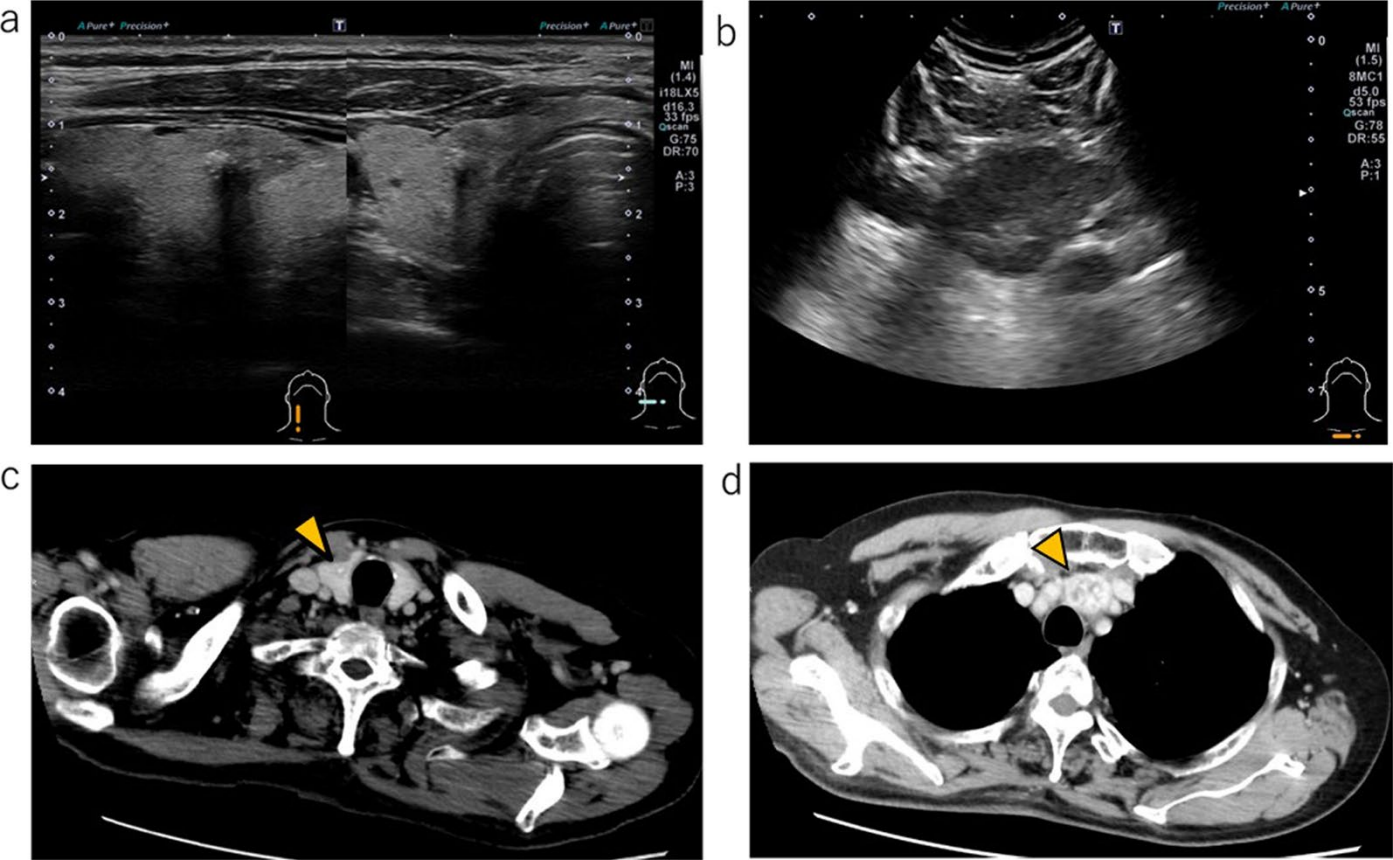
Preoperative ultrasonography and computed tomography (CT) scan. Ultrasonography revealed a hypoechoic nodule with internal calcification in the right thyroid lobe (a) and a hypoechoic mass in the superior mediastinum (b). CT scan showed a solid tumor with internal calcification in the right thyroid lobe (c) and a solid tumor with contrast enhancement in the superior mediastinum (d)
